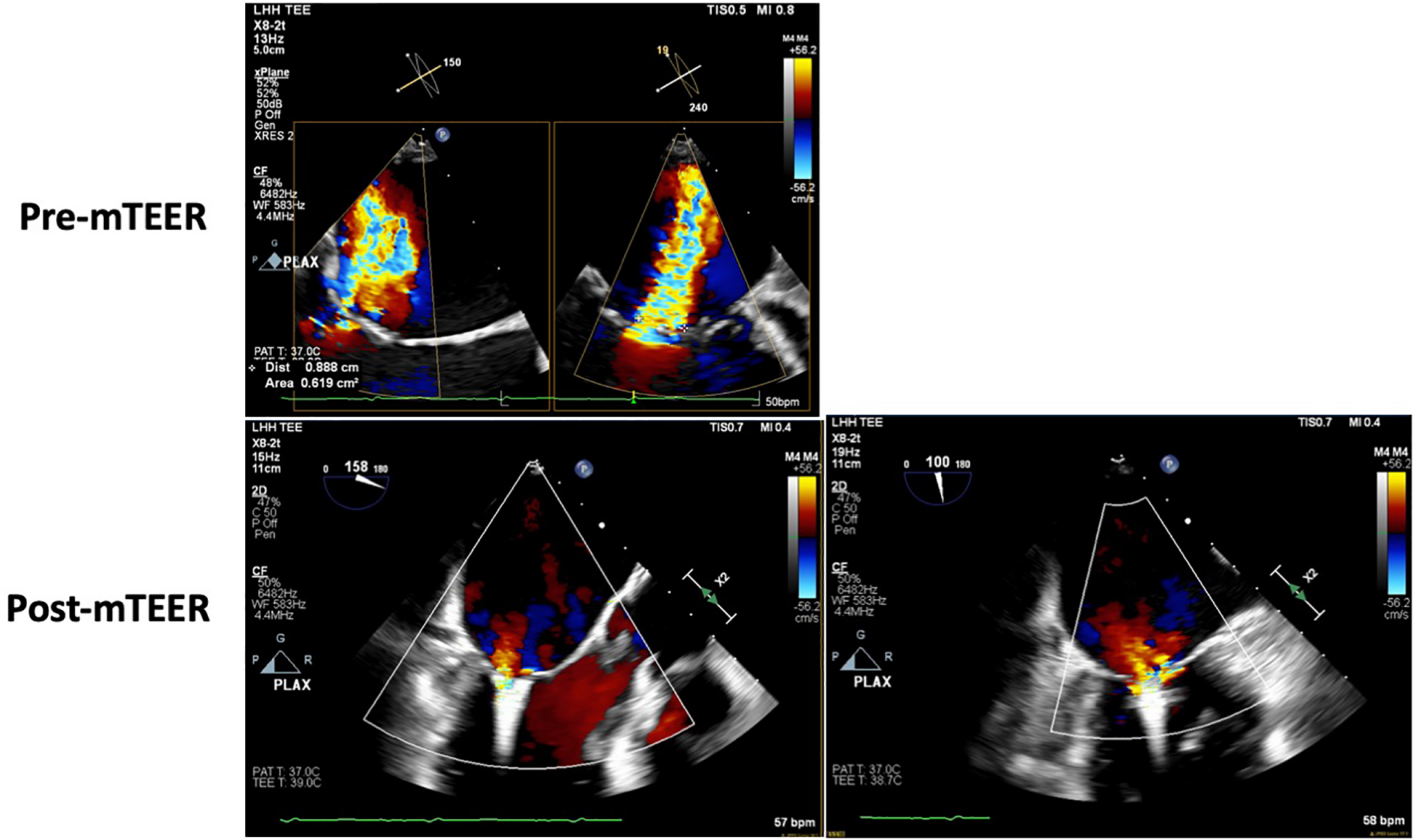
Case 5—transesophageal echocardiogram showing pre- and post-mitral TEER severity of mitral regurgitation.
